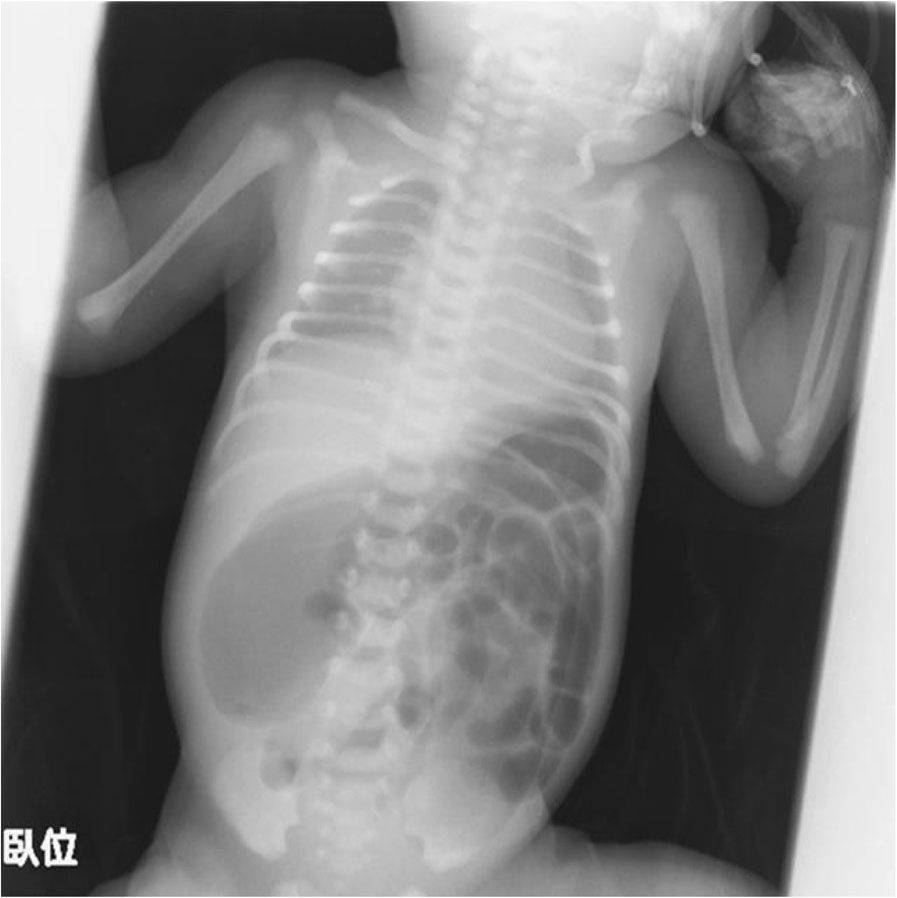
Plain abdominal radiography (36 h after birth). Plain abdominal radiography showed a significant dilation of the intestinal tract and no gas in the pelvic cavity
